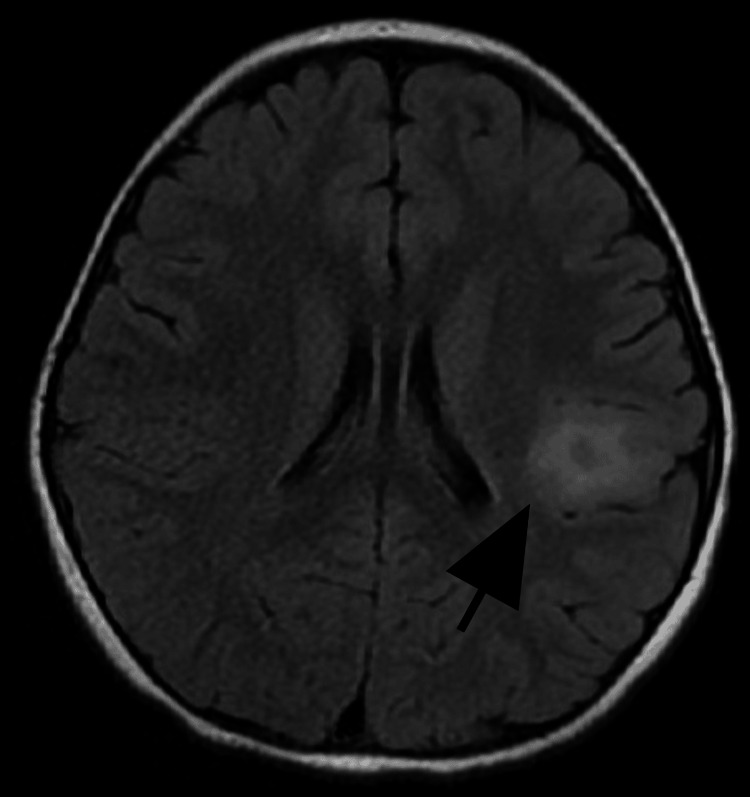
Brain MRI (axial FLAIR) Subtle T2/FLAIR hyperintensity in cortical grey matter. No diffusion restriction and normal sulcal/gyral patterns MRI, magnetic resonance imaging; FLAIR, fluid-attenuated inversion recovery
